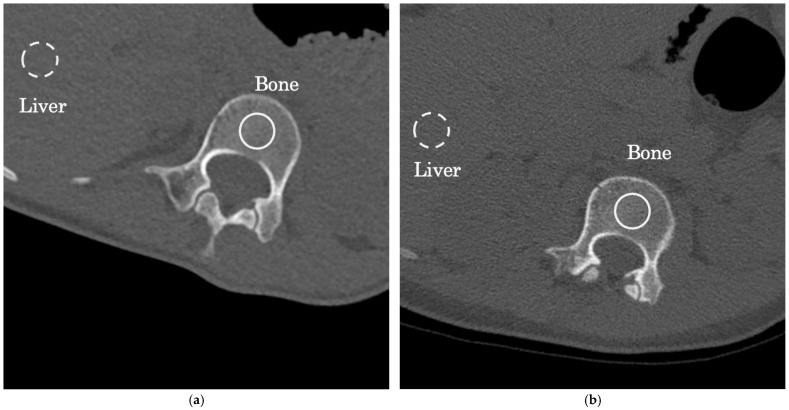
ROI (region of interest) setting points in the first lumbar vertebra (bone:) and liver for assessment of attenuation and image noise: (a) low-dose CT (LDCT); (b) normal-dose CT (NDCT).
